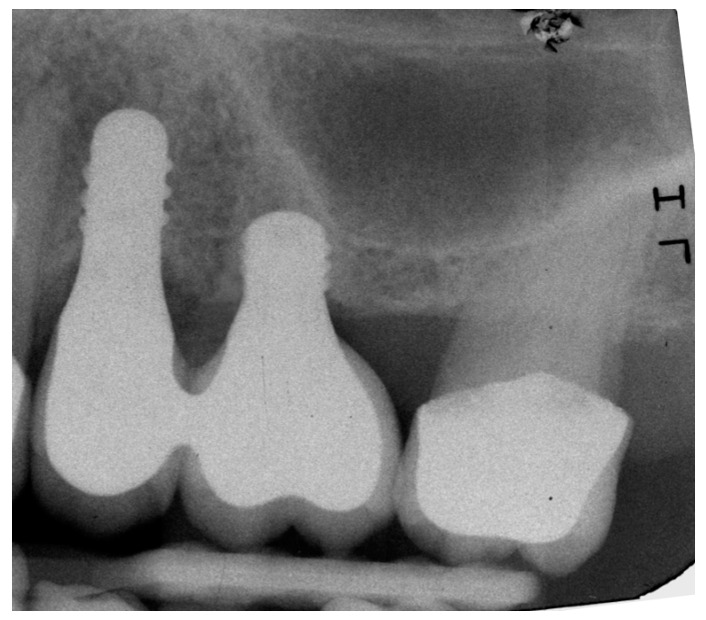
Radiograph of extra short and long implants at 24-month follow-up.
